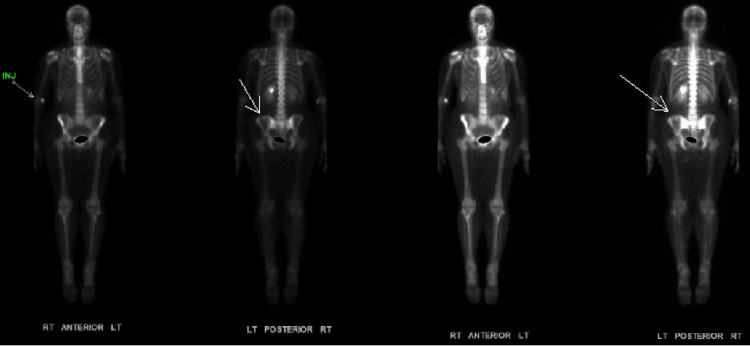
Bone scan with minimal radiotracer uptake in the left iliac wing (white arrows) corresponding to the initial CT image findings with injection site noted in the left antecubital fossa CT: computed tomography
